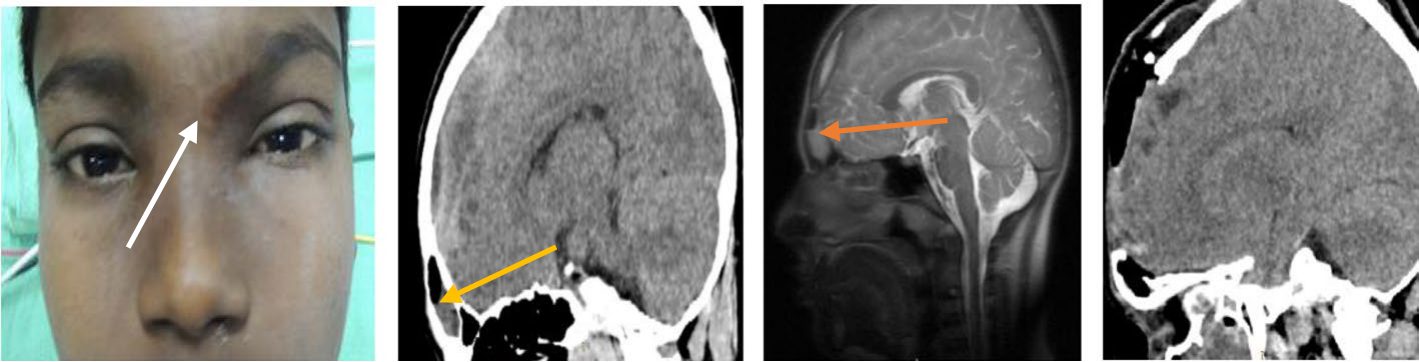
a–d Representative images of case series 1. a Clinical picture of the patient with a 2 × 1 cm tender swelling above (L) eyebrow. c (R) frontal sinus collection extending up to® frontal lobe with (R) frontal lobe abscess on CT scan. d Extradural collection in frontal region in midline with (R) frontal lobe abscess on MRI. e Post-surgery CT scan after wide bifrontal decompressive craniectomy
